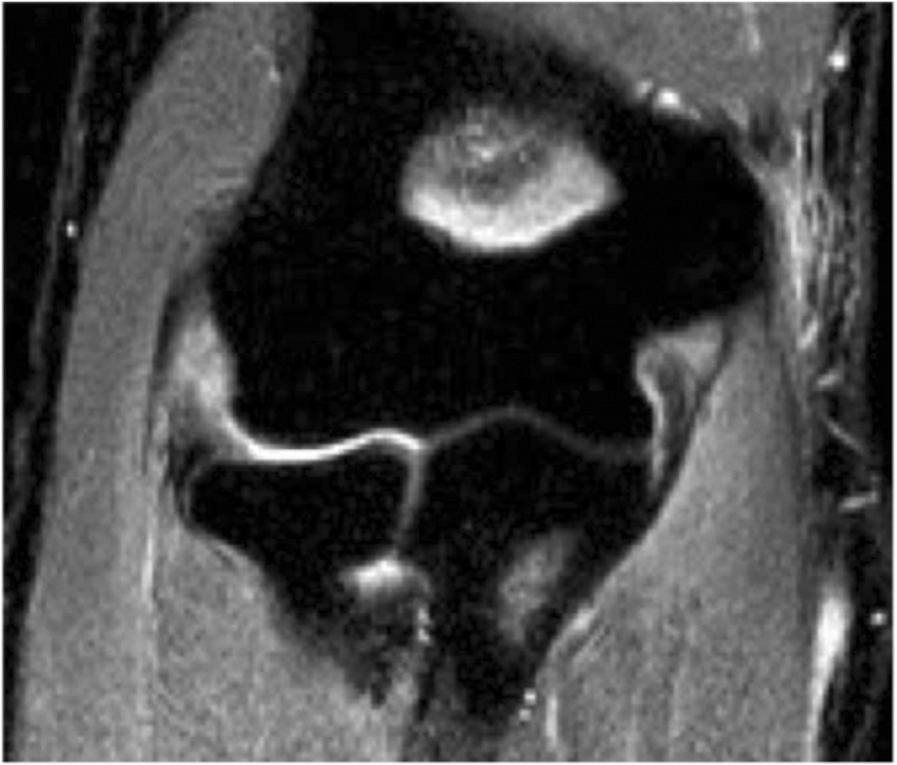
Example of a complete LCL tear and partial MCL tear in MRI
